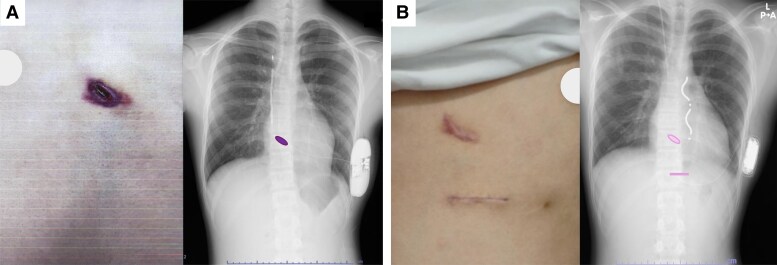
Pre- and post-procedural parasternal body surface appearances and chest radiographs in the case of EV-ICD implantation following S-ICD infection. An exposed subcutaneous lead at the midline infected incision site and a posteroanterior chest radiograph prior to the S-ICD extraction are shown (*A*). A post-operative picture of the parasternal body surface appearance and a radiograph after 1 month showed successful EV-ICD implantation with a sufficient distance between the previous and the newly created incisions (*B*). EV-ICD, extravascular implantable cardioverter-defibrillator; S-ICD, subcutaneous implantable cardioverter-defibrillator.
